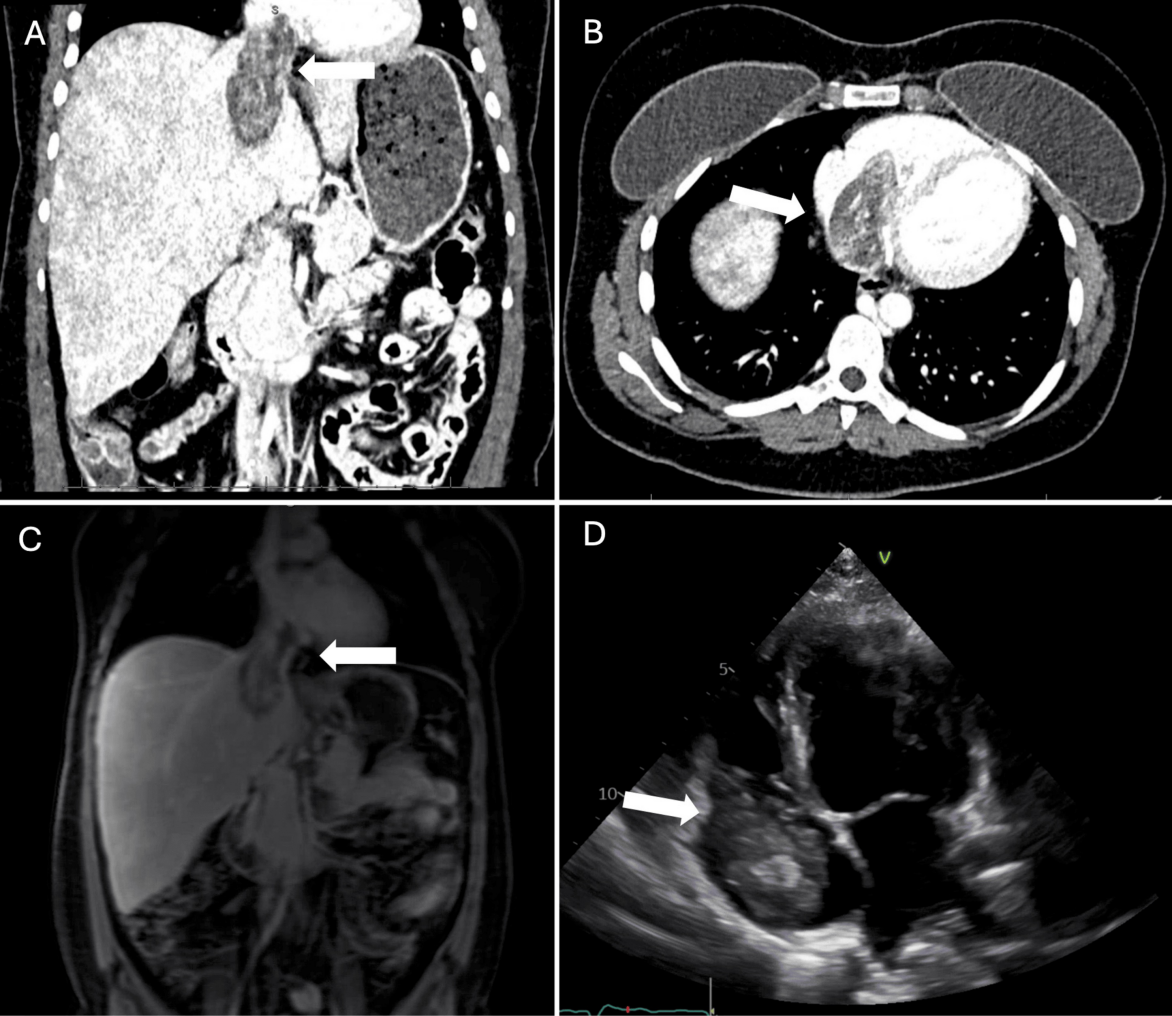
Preoperative CT, MRI, and TTE Figures 1A, 1B: CT abdomen with contrast: a mass in the IVC extending to the RA (white arrow). 1C: A mass 3.4 x 3.4 x 7.0 cm in size extending from the IVC to RA without evidence of focal hepatic lesion or renal mass (white arrow). 1D: TTE: A large mobile mass or thrombus in the RA that was prolapsed across the tricuspid valve into the right ventricle (white arrow)
